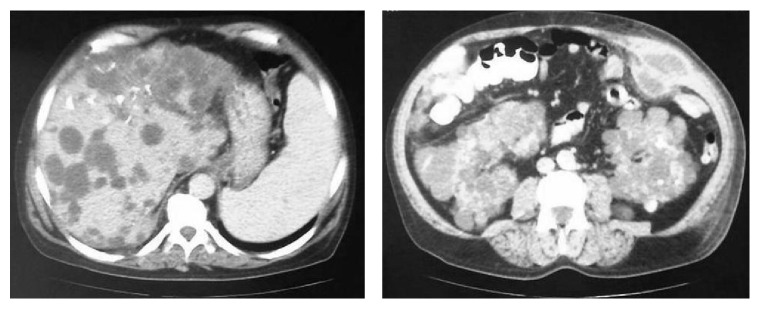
Abdominal Computed Tomography: diffuse involvement of polycystic disease in both kidneys, liver, and colon.
